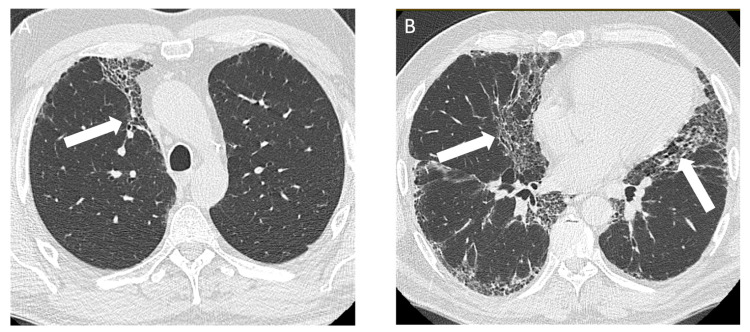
A 56-year-old male with a remote 20 pack year smoking history and a history of beryllium exposure who was working with electronics, diagnosed with chronic beryllium disease. Axial computed tomography shows interstitial fibrosis characterized by (A) peripheral subpleural and (B) peribronchovascular distribution of reticulation and mild traction bronchiectasis (arrows) with a basal predominance.
